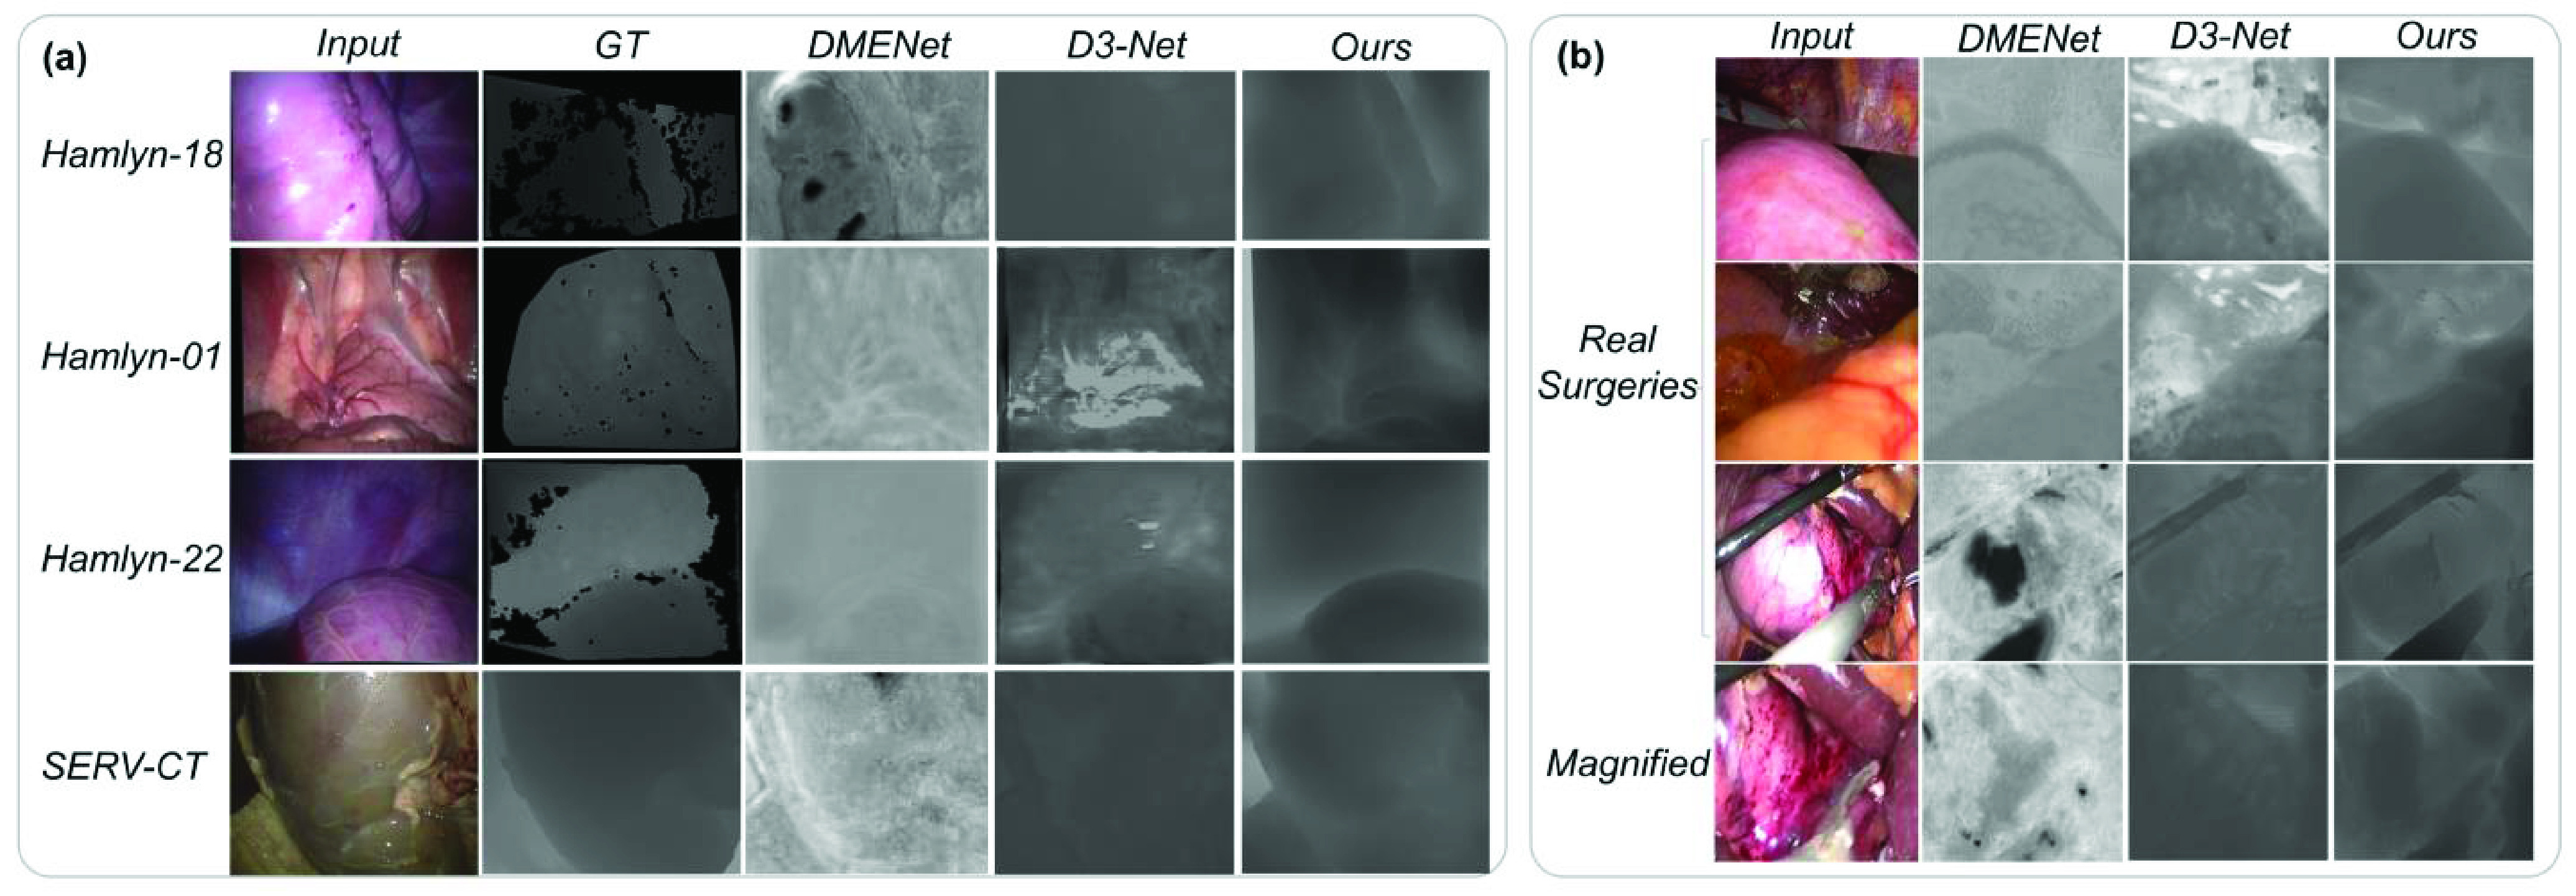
(a) Depth outputs of DMENet [27], D3-Net [29] and our proposed MDFNet on the Hamlyn dataset no.18, no.1, no.22, SERV-CT dataset, (b)real defocused and in-focus clinical surgeries images provided from Changgung Hospital real defocused and in-focus clinical surgeries images provided from Changgung Hospital using a Storz laparoscope (patients suffering from hepatolithiasis which need minimally invasive hepatectomy), with the last row a magnified view of the clinical scene, used to show our depth estimation of magnified scenes help improve the depth resolution.
